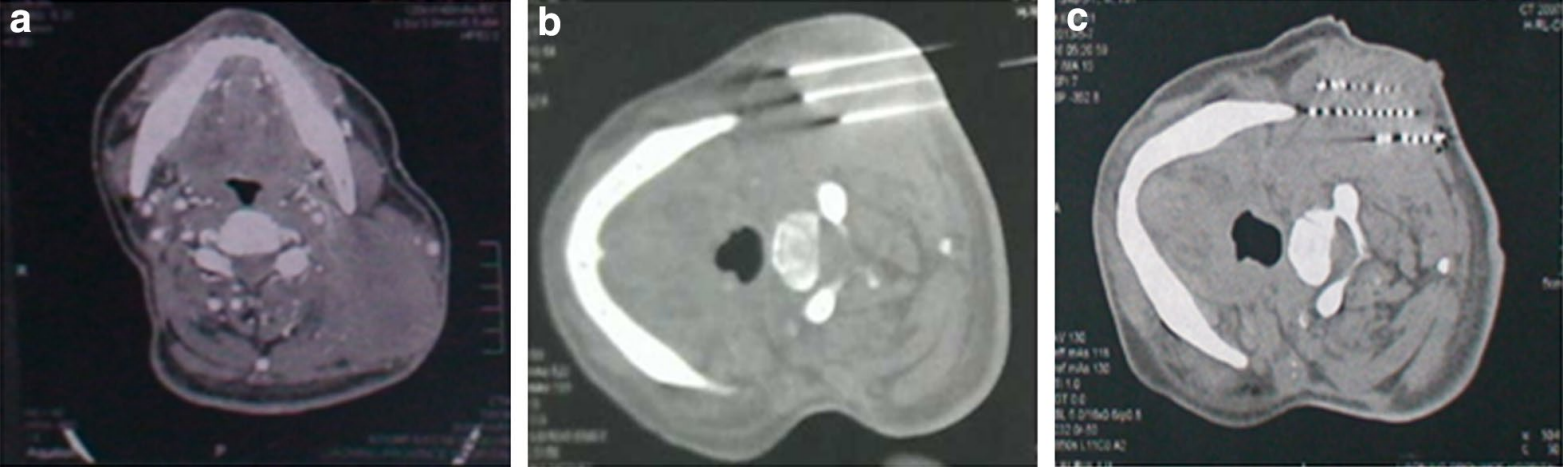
Treatment of a patient with cervical lymph node metastasis in primary nasopharyngeal carcinoma. a CT scan shows left neck lymph node metastasis before implantation. b CT scan shows that ^125^I seeds are implanted into the tumor via implantation needles. c CT scan shows that ^125^I seeds are implanted post-implantation 3 months. *CT* computed tomography
